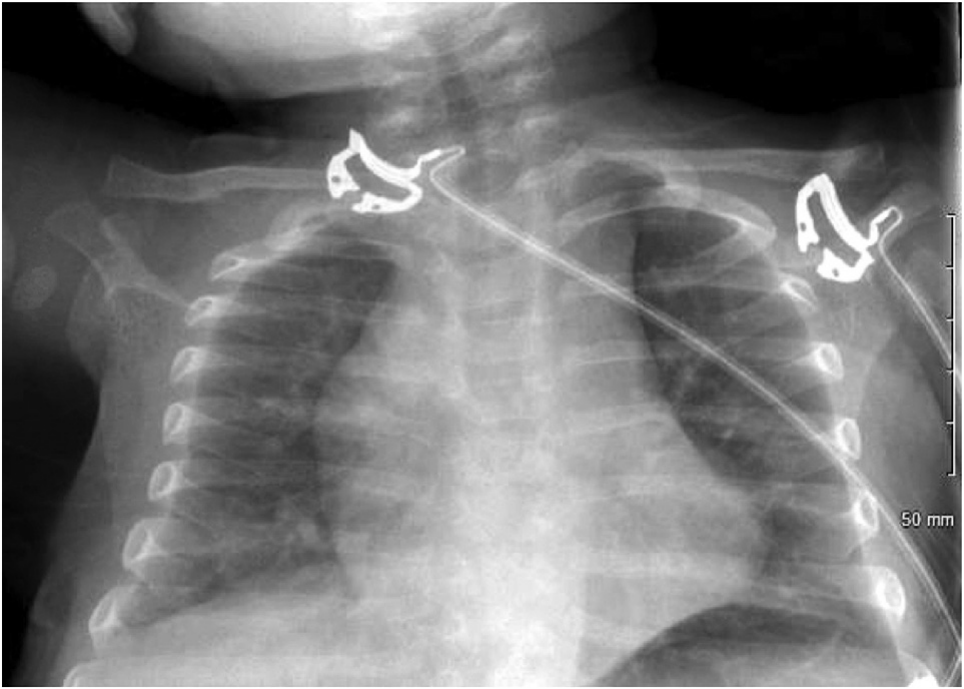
Chest radiograph from initial evaluation in the emergency department, without evidence of pneumonia.
